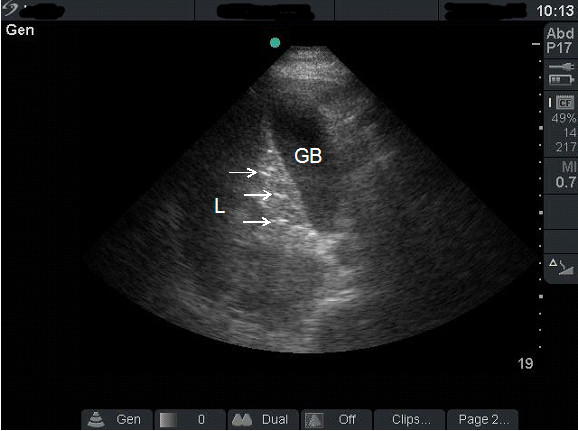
Transverse sonographic section of the right upper quadrant using a curvilinear probe showing hyperdence echogenic small areas (arrows) between the gall bladder (GB) and the liver (L) indicating free air.
